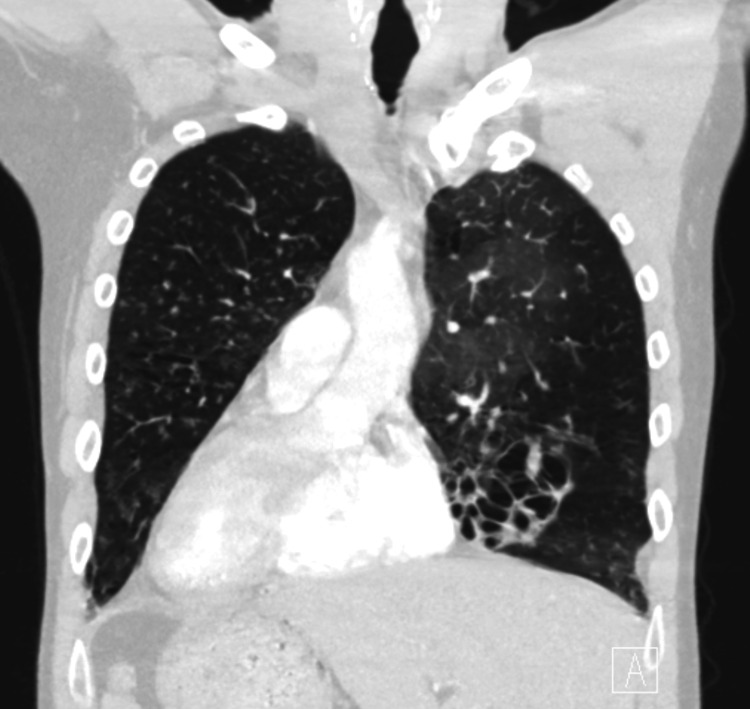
CT scan showing dextrocardia and bronchiectasis
